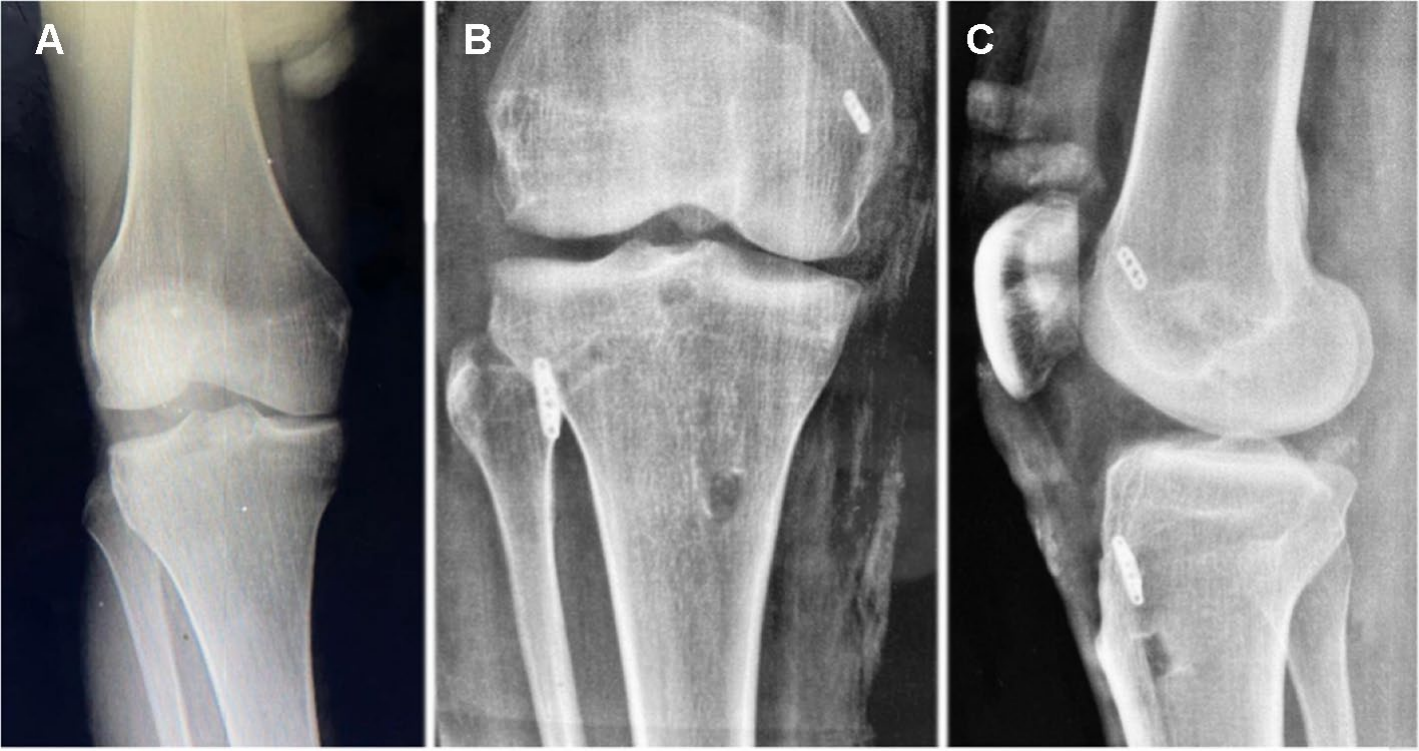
A Preoperative varus-stress radiograph of a patient with an acute combined PLC + PCL injury. B 1-yeay postoperative varus-stress radiograph with significant reduction of the lateral joint line opening to the normal contralateral value. C Lateral X-ray showing the position of the adjustable button at the anterolateral tibial surface. *PLC* posterolateral corner, *PCL* posterior cruciate ligament
